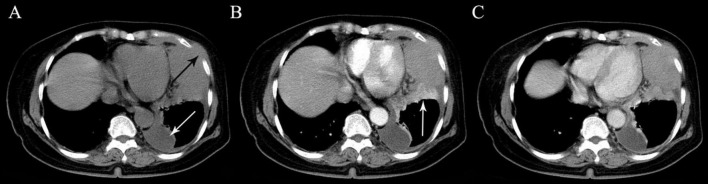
A 71-year-old woman with malignant pleural mesothelioma. (A) A solid mass with irregular shape is seen in the left lower thoracic cavity on precontrast CT. Intercostal soft tissue invasion (black arrow) and pleural effusion (white arrow) are also seen. (B) Segmental atelectasis with marked enhancement in the lower lobe of the left lung is detected in the arterial phase (arrow). (C) The tumor shows heterogeneous mild to moderate enhancement in the venous phase.
